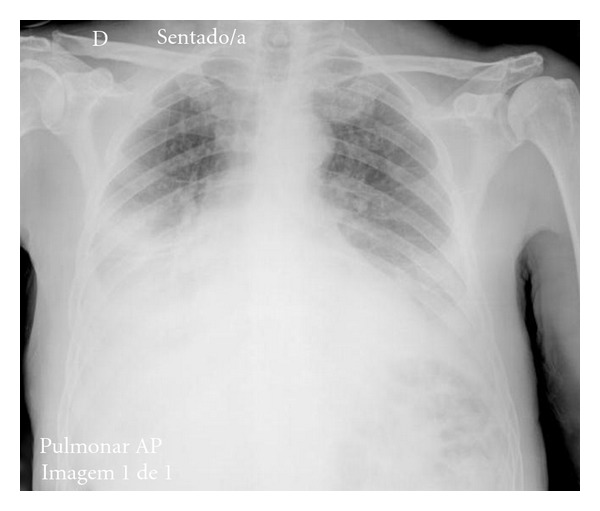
Chest X-ray on the day of admission.
